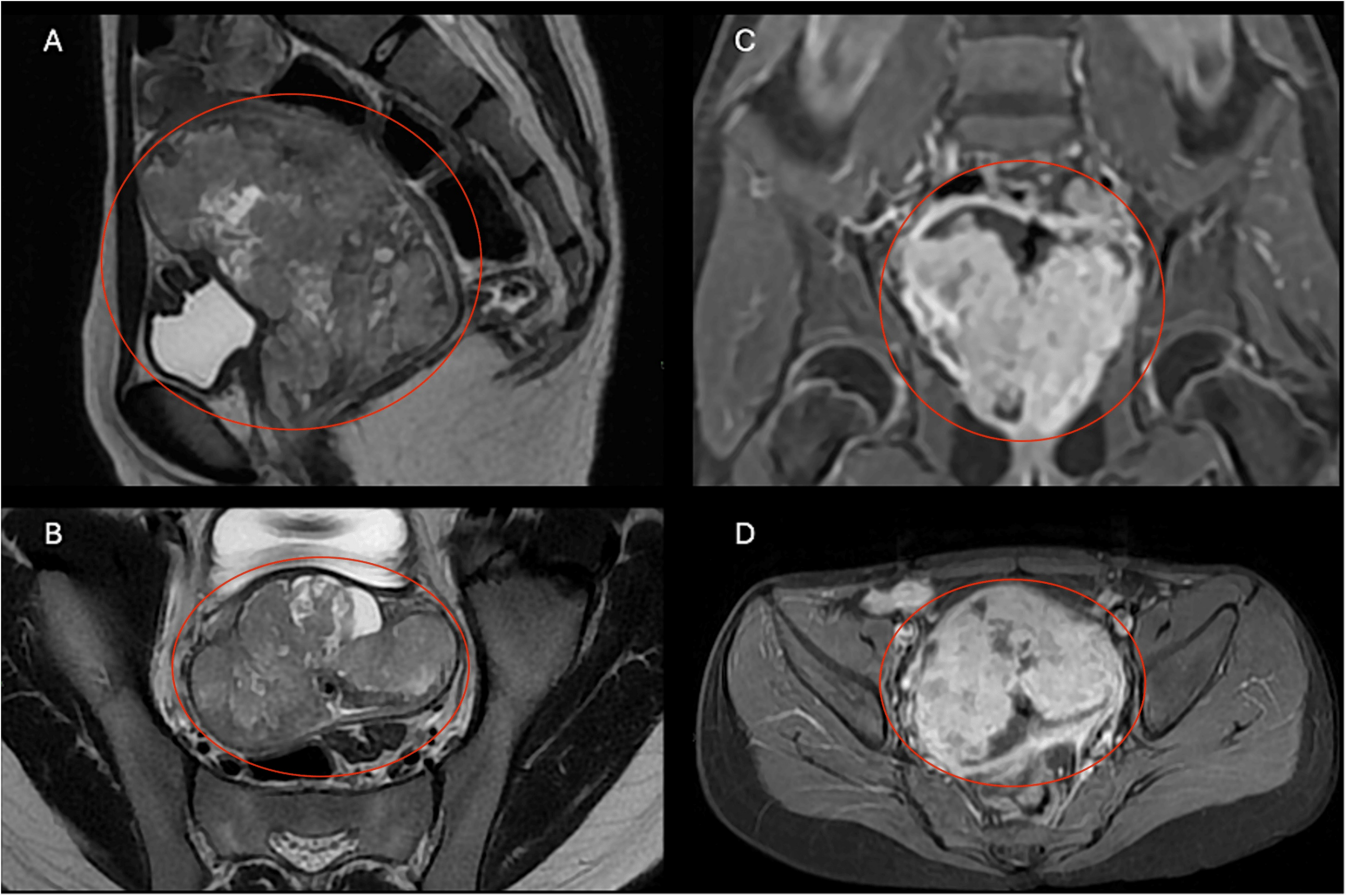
(A, B) T2-weighted sagittal and axial MRI showing a heterogeneous mass (red circles) occupying and distending the vaginal cavity without extension to adjacent structures; (C, D) T1-weighted fat-suppressed coronal and axial images demonstrating hypervascular enhancement of the vaginal lesion (red circles)
